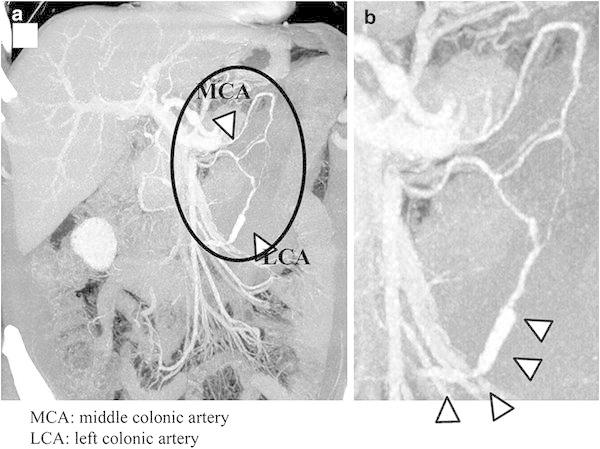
a Maximum-intensity-projection (MIP) image from contrast-enhanced coronal CT angiography. b Extended figure of part of the circulation in (a) demonstrates intermittent arterial dilatation like fusiform aneurysms (*arrow*) in the left colonic artery
